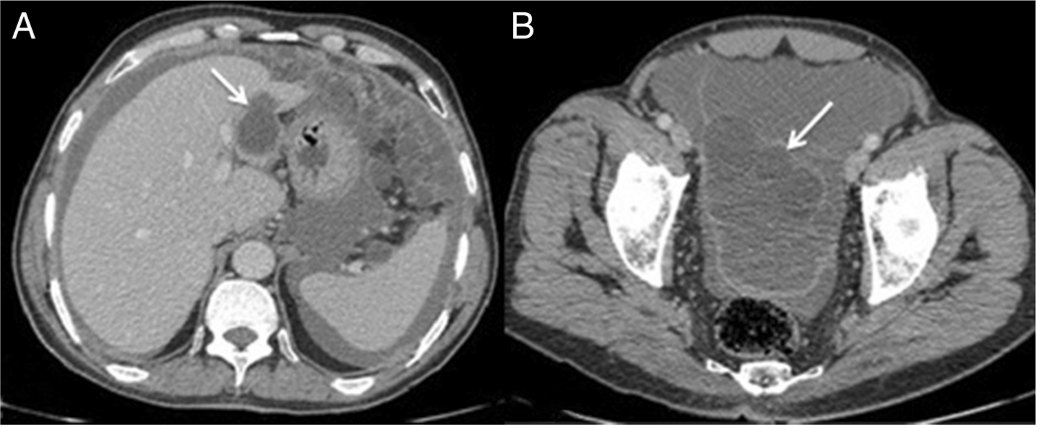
Axial contrast-enhanced CT scan. A. Well-defined and thin walled cystic lesion in the segment II of the liver (Arrow). B. Widespread ascites and cysts into the peritoneal cavity (Arrow).
